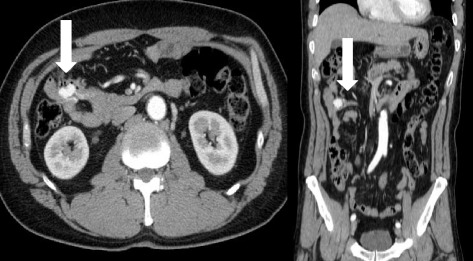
Abdominal enhanced computed tomography showing a 2-cm hypervascular tumor in the small intestine
